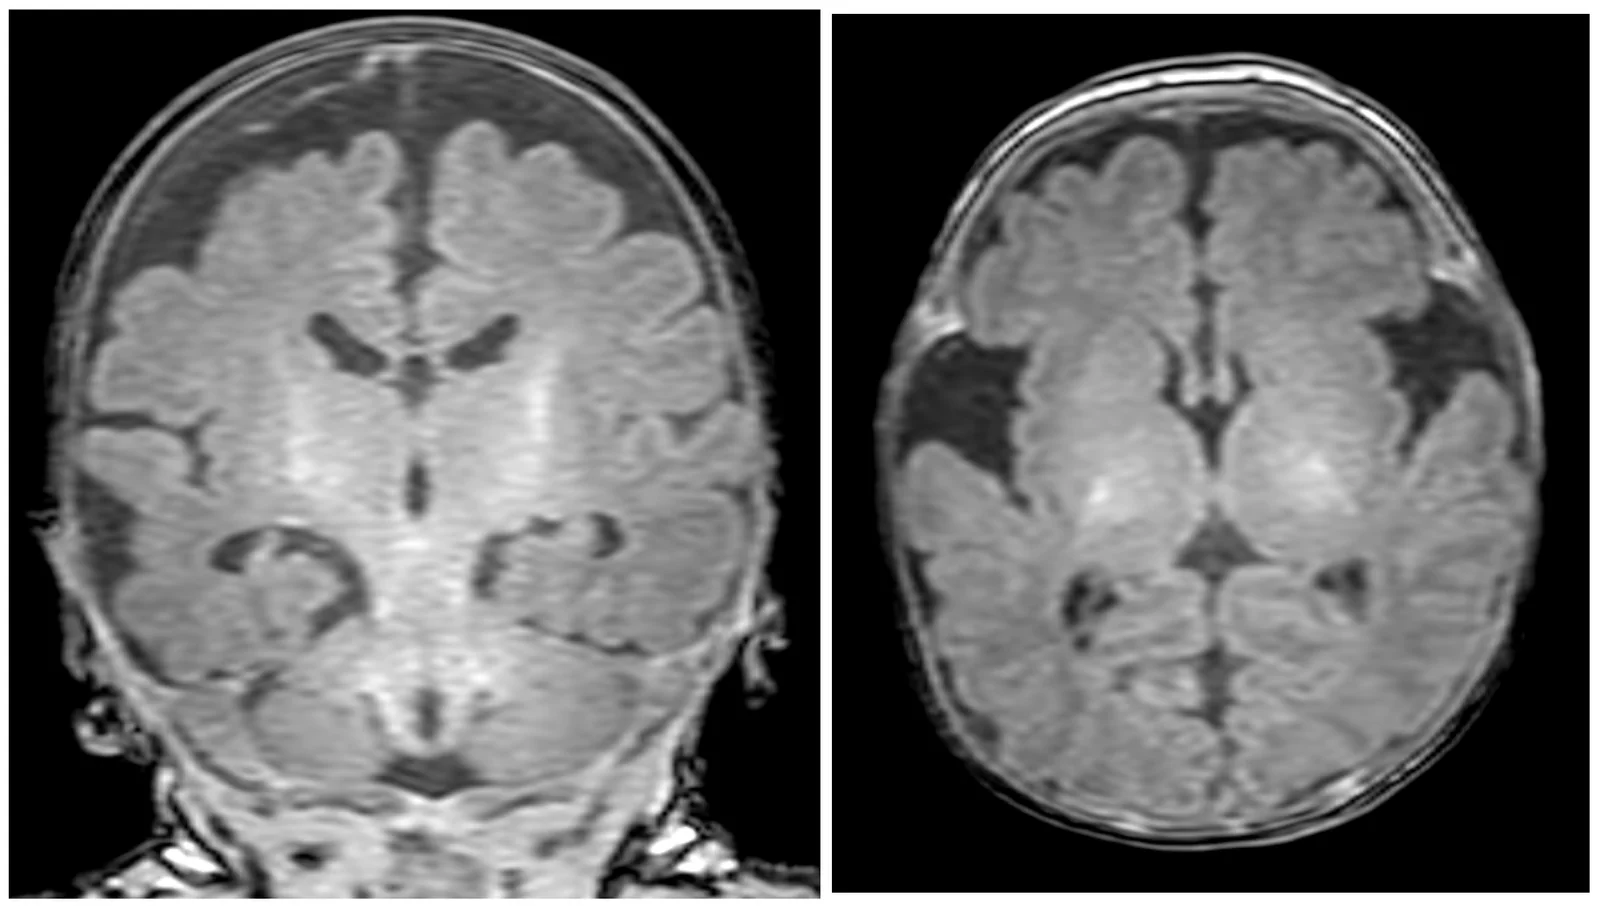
Preterm birth has been shown to be associated with prominent extra-axial spaces, thinning of the corpus callosum, and under-opercularization of the sylvian fissures, as demonstarted in these coronal T1-weighted (left) and axial T1-weighted images (right). Images are original and obtained from Rady Children’s Hospital’s deidentified patient cases.
